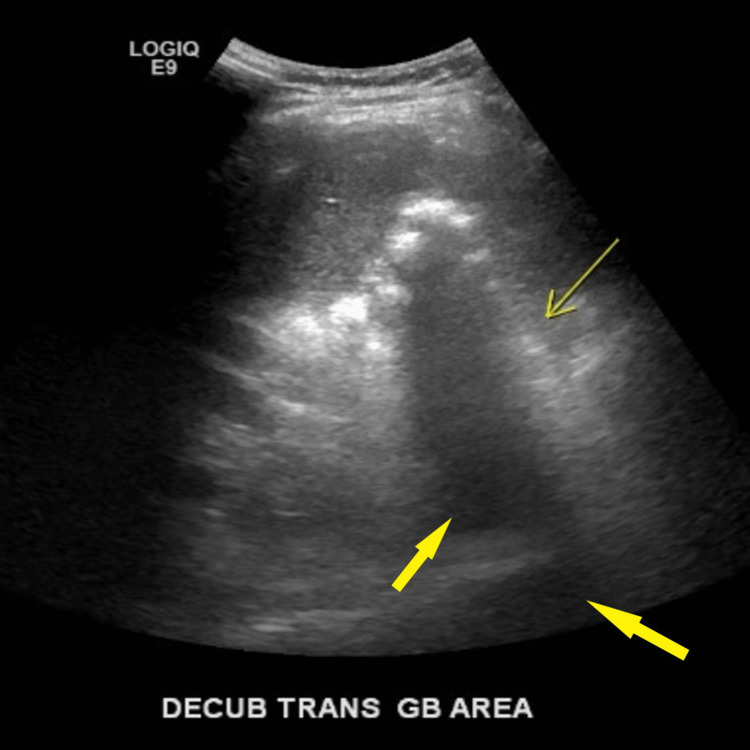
An ultrasound of the right upper quadrant reveals gallstones and shadowing. Shown is a transabdominal decubitus view of the gallbladder area. Thick yellow arrows indicate shadowing, consistent with gallstones. The thin yellow arrow indicates the gallbladder area.
